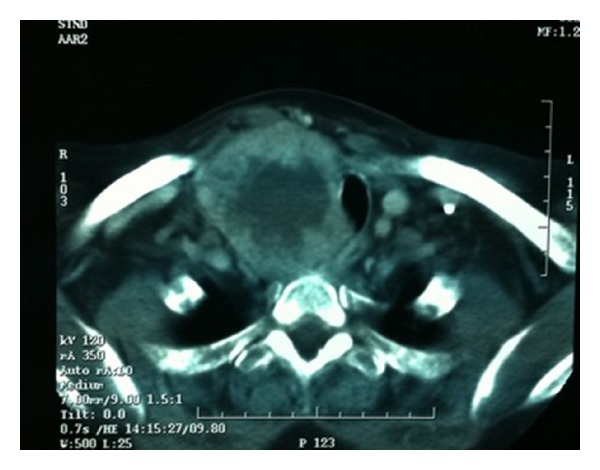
CT scan showing the mass pushing the trachea and occluding its lumen.
